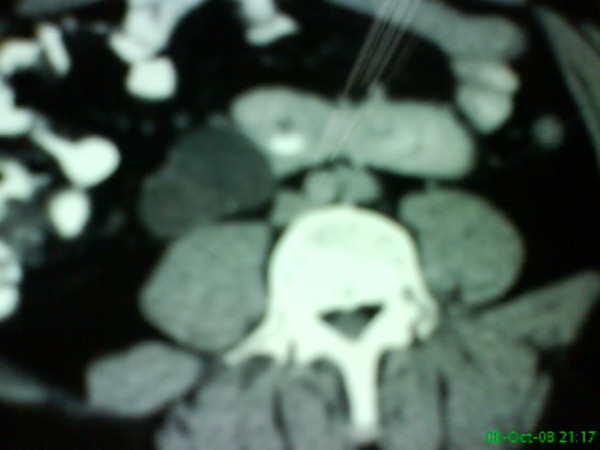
CT scan showing isthmic stone in a horseshoe kidney.
